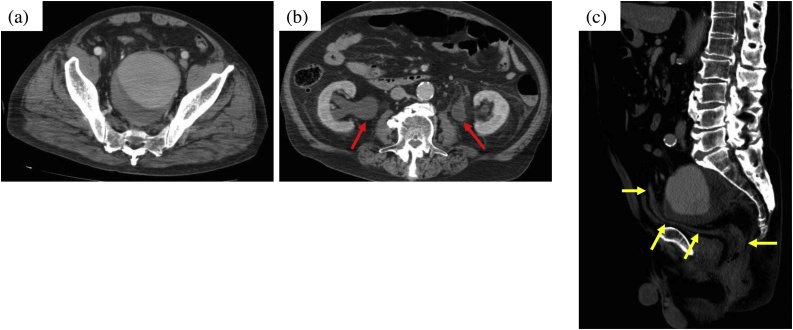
Initial contrast-enhanced CT findings. (a) An isolated internal iliac artery aneurysm (IIAA; maximum diameter, 90 mm) occupying the pelvic cavity. (b) Bilateral hydronephrosis is observed (red arrows), suggesting ureteral obstruction due to the IIAA. (c) The rectum is compressed by the aneurysm, resulting in a narrowing (yellow arrows indicate rectum).
